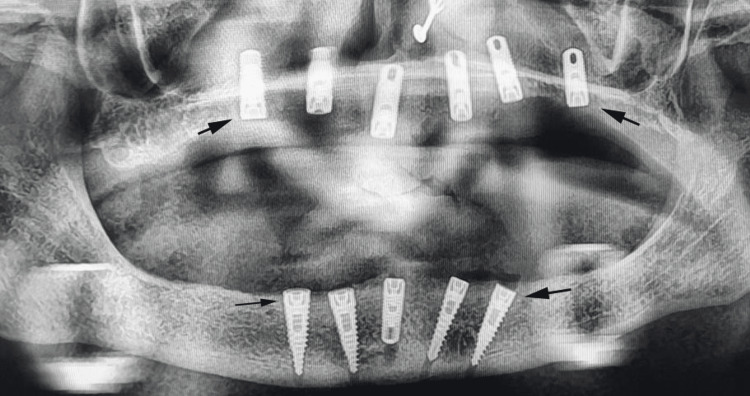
Orthopantomogram showing edentulous maxillary and mandibular jaw with multiple implants (black arrows) placed for full-mouth rehabilitation Original image from study data.
